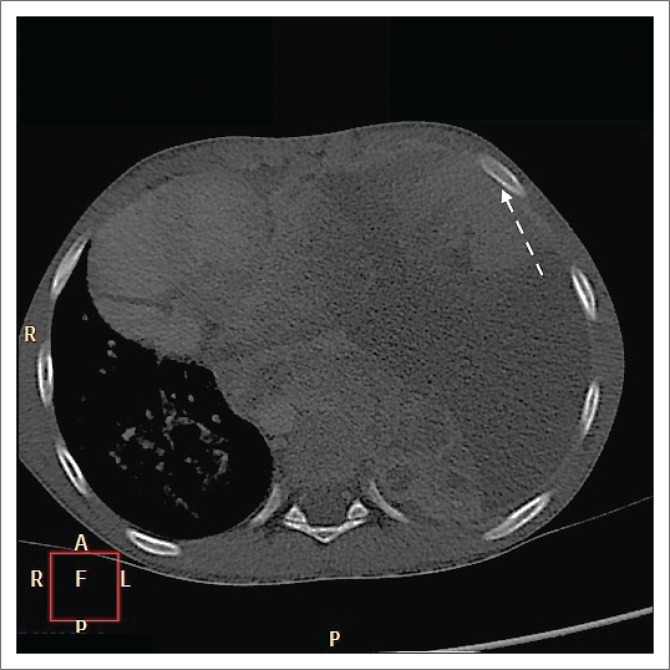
Axial computed tomography image of the chest on bone window demonstrates the absence of associated bony erosions (white arrow).
